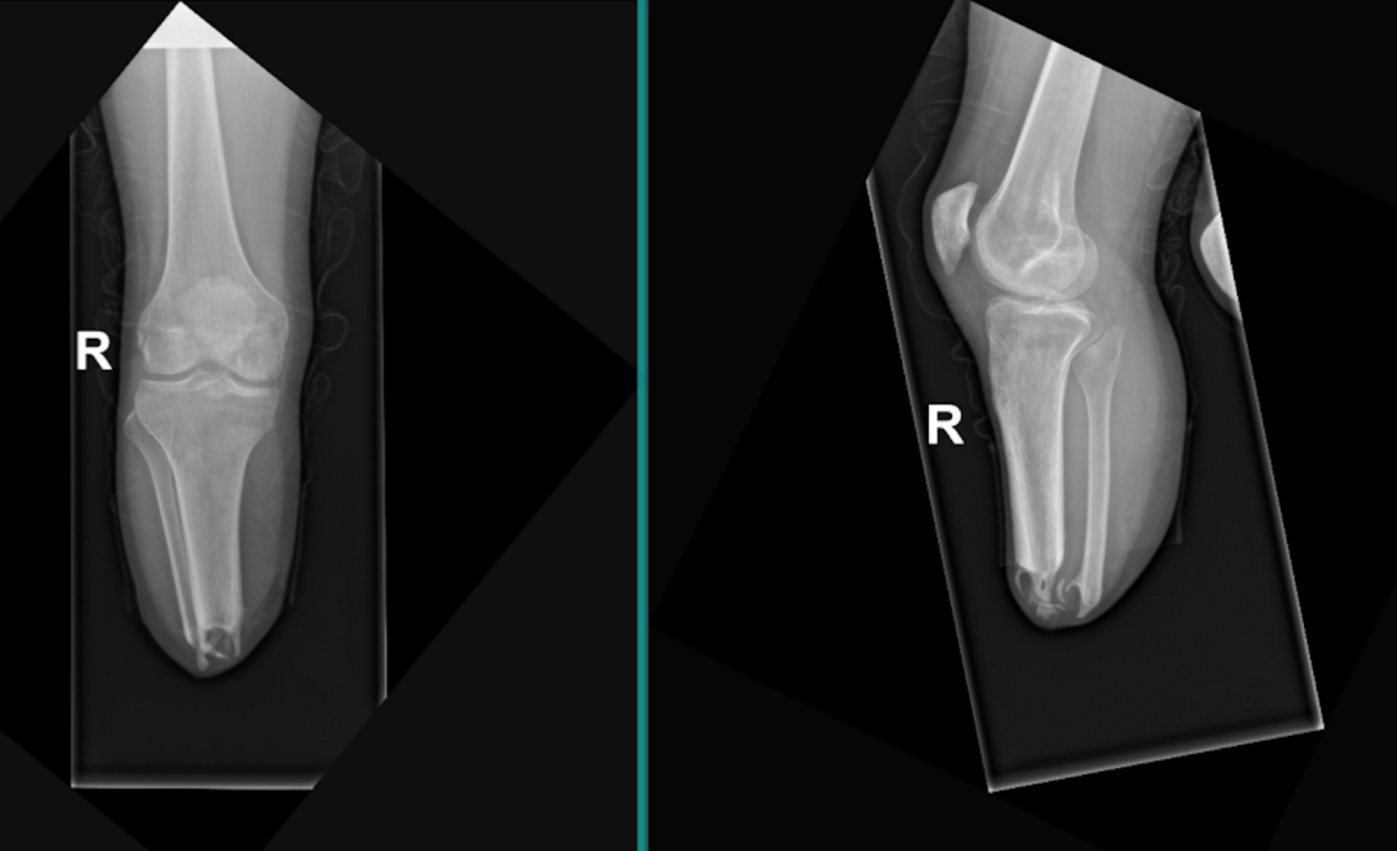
Right lower extremity anteroposterior (left) and lateral (right) plain radiograph of transtibial amputation with distal heterotopic ossification of the tibia and fibula stumps.
